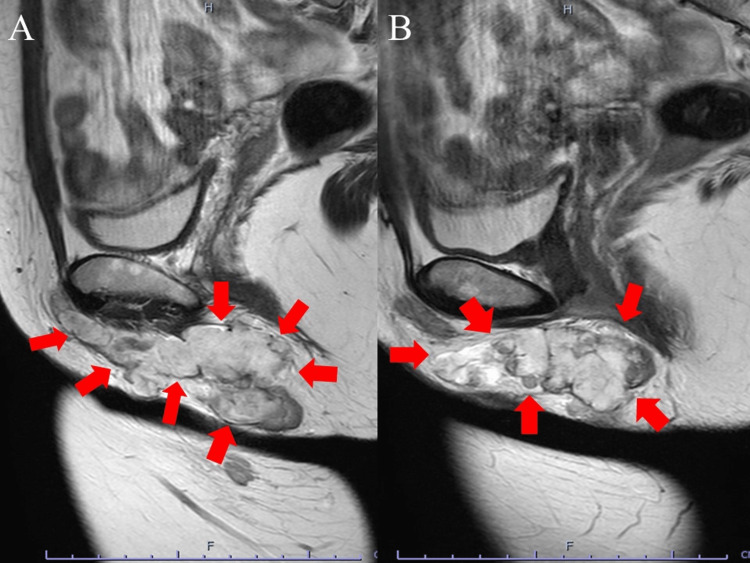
Magnetic resonance images. Sagittal section of T2-weighted image. T2-weighted image shows a hyperintense signal. The image in B is more on the central side than in A.
